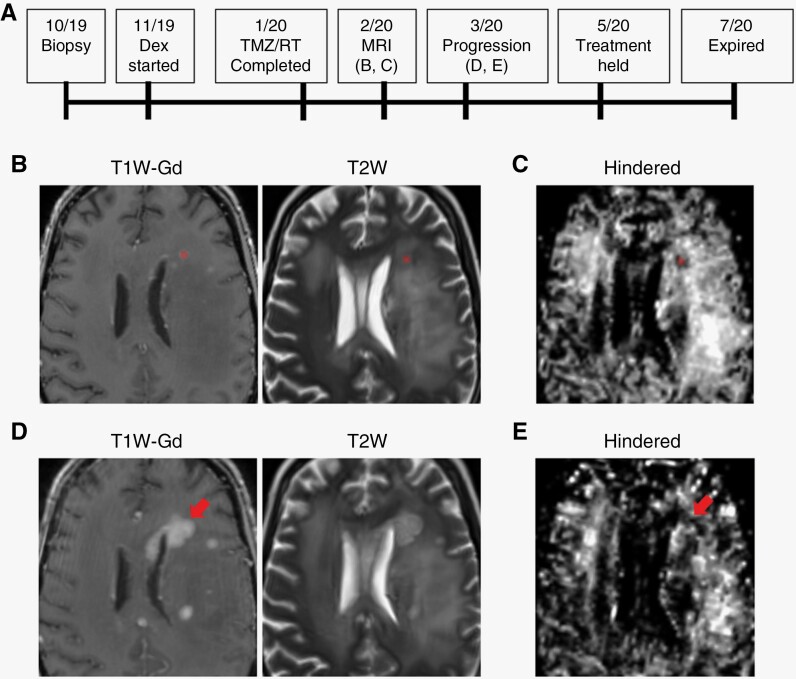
Serial imaging for a case of true tumor progression. (A) Treatment timeline for a 40-year-old female patient with diffuse astrocytic glioma with molecular features of glioblastoma, CNS WHO grade 4, indicating timing of panels B to E. (B) Post-radiotherapy MRI showed interval increased size of T2 signal abnormalities and new enhancing lesions. (C) DBSI hindered fraction map on the same date as (B) showed hypointensity in the regions of new contrast enhancement, which would have suggested disease progression. Segmented regions used in quantitative analyses are “highlighted”. (D) Two-month follow-up MRI confirmed tumor progression due to enlargement and interval development of new multifocal enhancing nodules with worsening T2 hyperintensity. (E) Follow-up DBSI hindered fraction map showed enlarging hypointensity in the same regions as (D) consistent with progression. Dex, dexamethasone; TMZ, temozolomide; RT, radiotherapy; T1W-Gd, T1-weighted post-gadolinium; T2W, T2-weighted.
